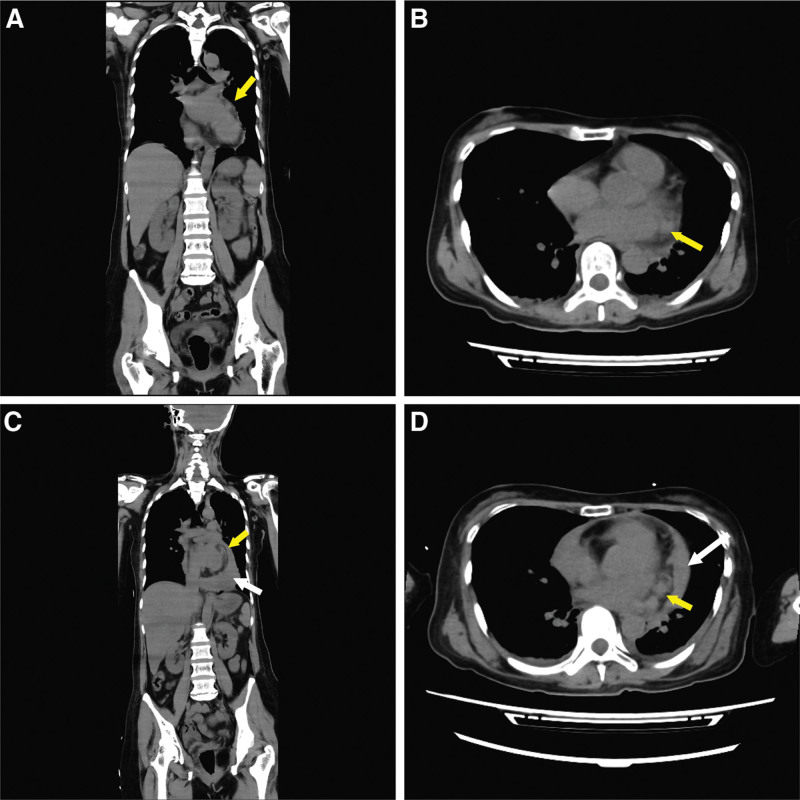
A. Preoperative computed tomography (CT) scan taken at admission. The preoperative sagittal CT image shows a mass in the pericardial space at the posterior wall of the left ventricle (yellow arrow). B. Preoperative CT scan taken at admission. The preoperative horizontal CT image shows a mass in the pericardial space at the lateral wall of the left atrium and below the left atrial appendage (yellow arrow). C. Preoperative CT scan taken after cardiopulmonary resuscitation. The preoperative sagittal CT image after cardiopulmonary resuscitation shows a mass in the pericardial space on the posterior wall of the left ventricle (yellow arrow) and pleural effusion (white arrow). D. Preoperative CT scan taken after cardiopulmonary resuscitation. The preoperative horizontal CT image after cardiopulmonary resuscitation shows a mass in the pericardial space at the posterior wall of the left ventricle (yellow arrow) and pleural effusion (white arrow).
